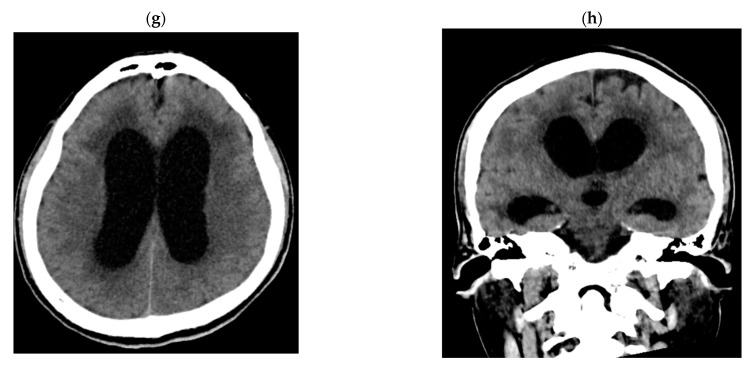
Images of case 1: (a) FLAIR and (b) T1 with gadolinium show nodular hyperintensities in the basal ganglia, with irregular annular enhancement. Case 5 images: T1-weighted images (c) show irregular leptomeningeal enhancement with (d) underlying parenchymal edema observed on FLAIR. Case 7 images: (e,f) Cerebral tomography showing subacute left parietal and occipital infarction, characterized by cortical and subcortical hypodensity. Case 8 images: (g,h) Tomography showing acute hydrocephalus with transependymal edema.
